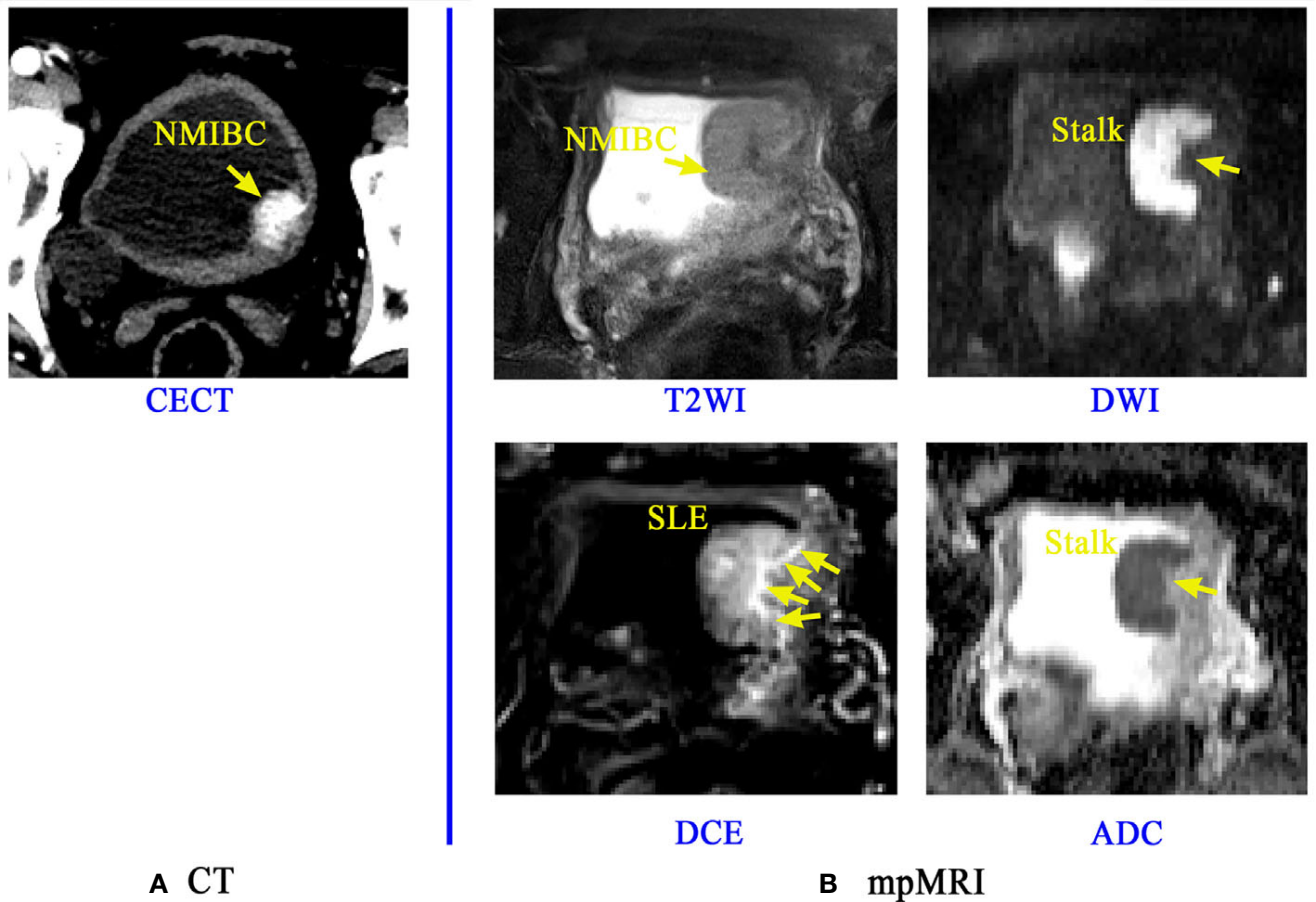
Application of CT and mpMRI for the preoperative prediction of the muscle invasion status of BCa. A lesion of a patient confirmed with NMIBC is discernible on Contrast-enhanced CT (CECT) image (A), but the boundaries and basal part of this lesion is rarely distinguishable. The mpMRI (B) including the T2WI, DCE, DWI and its corresponding ADC map can provide more important signs and information like the stalk at the tumor base and submucosal linear enhancement (SLE) for accurate diagnosis of muscle-invasive status (MIS) of BCa (38).
